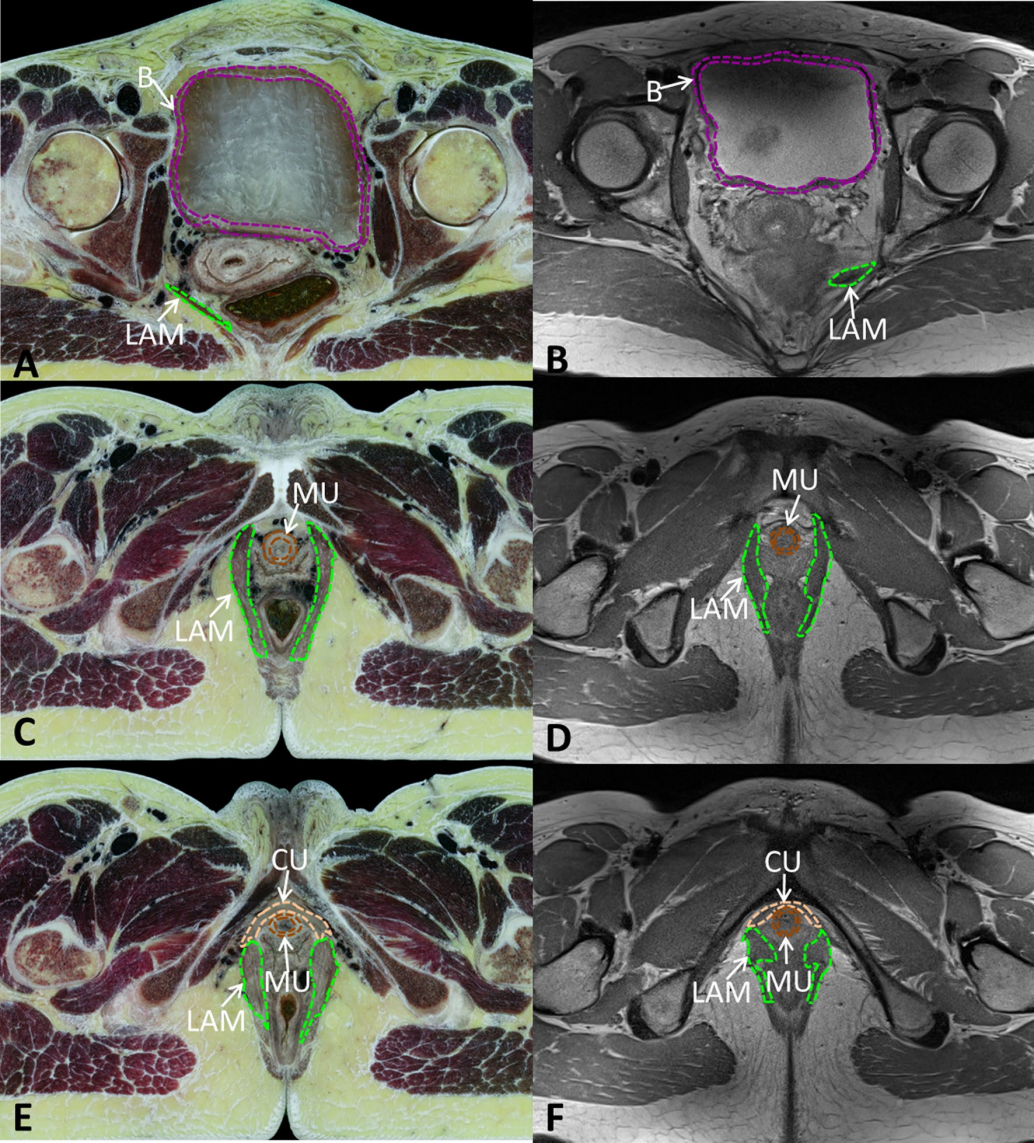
Comparison and segmentation between CVH and MRI pelvic floor. A, B Comparison and segmentation between CVH and MRI in bladder section; C, D Comparison and segmentation between CVH and MRI in superior urethra section; E, F Comparison and segmentation between CVH and MRI in inferior urethra section. CVH, Chinese Visible Human; LAM, levator ani muscle; B, bladder detrusor; MU, main part of urethral sphincter; CU, compressor urethrae
